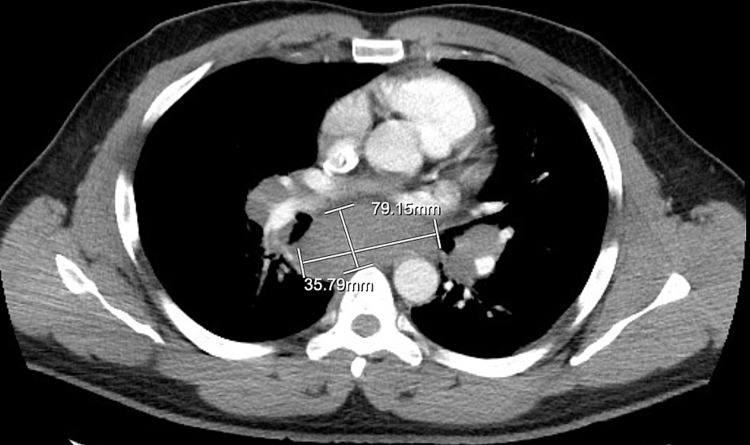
CT chest without contrast Multiple arch mediastinal and bilateral hilar lymph nodes, including a subcarinal lymph node conglomerate measuring 7.9 x 3.6 cm.
